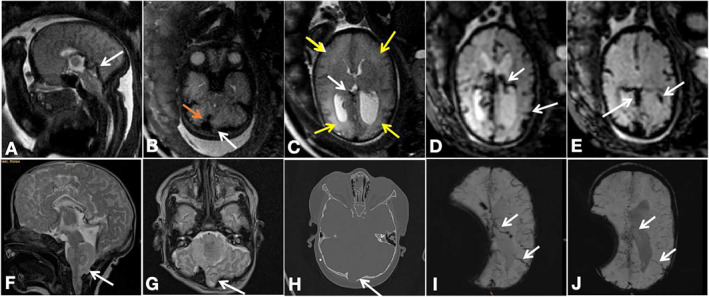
Phenotype of Chiari II malformation with brain edema, venous congestion, and intracranial hemorrhage. (A–E) Prenatal MRI performed at 35 + 3 weeks. (A) T2‐weighted images show a dilated straight sinus. (B) The herniating cerebellum protruding posteriorly to the occiput bone, the transverse sinus is compressed and narrowed (white arrow), and the adjacent dilated torcular (orange arrow) was noted. (C) Brain parenchyma edema (yellow arrows) and congestion in the deep ventricular veins (white arrow) were identified. (D, E) EPI‐T2* images show congested internal cerebral vein, superficial cortical veins, and ependymal hemorrhage at the left posterior horn (white arrows). (F–J) Postnatal MRI (after postnatal MMC repair surgery and EVD shunt) also demonstrates the protruding cerebellum (F, G) and the adjacent skull thinning like a notch shown on CT (H). (I, J) Follow‐up postnatal MRI performed at 3 weeks of age shows the congested cortical veins and deep veins at first (E, white arrow) and then, released at a subsequent scan performed at 23 months of age (F).
